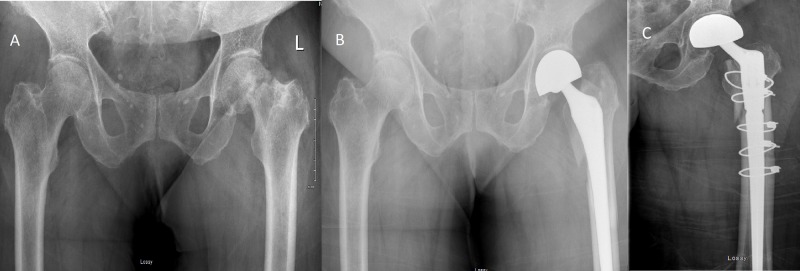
Plain anterior-posterior (AP-PA) radiographs showing initial pathological fracture (A), first surgical intervention with early periprosthetic fracture (B) and result of revision surgery (C).
